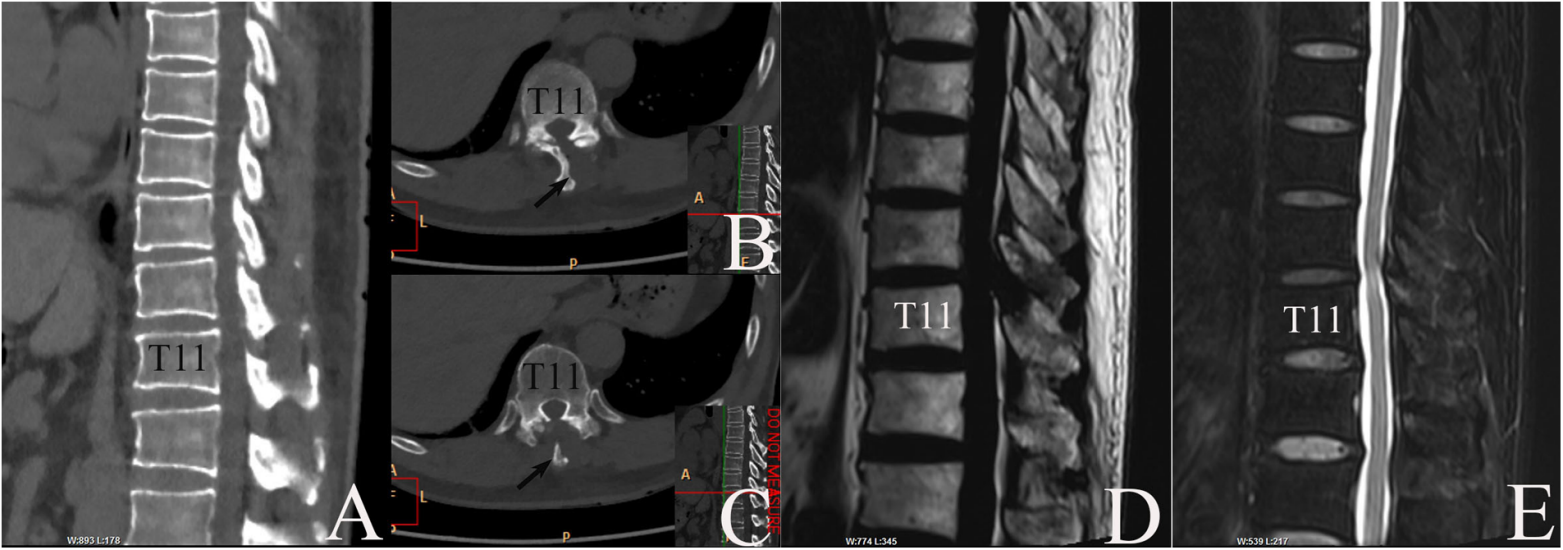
MR and CT re-examinations postoperatively. (A–C) CT taken on 15 May 2019 confirmed disappearance of CLF and fenestration at left T10–11 interlaminar space. (A) Midsagittal plane, bone window. (B, C) Axial plane, bone window. Arrow: T10 spinal process. (D, E) MRI taken on 26 December 2019 revealed complete recovery of dura sac. (D) Midsagittal T1WI image. (E) Midsagittal T2WI image.
